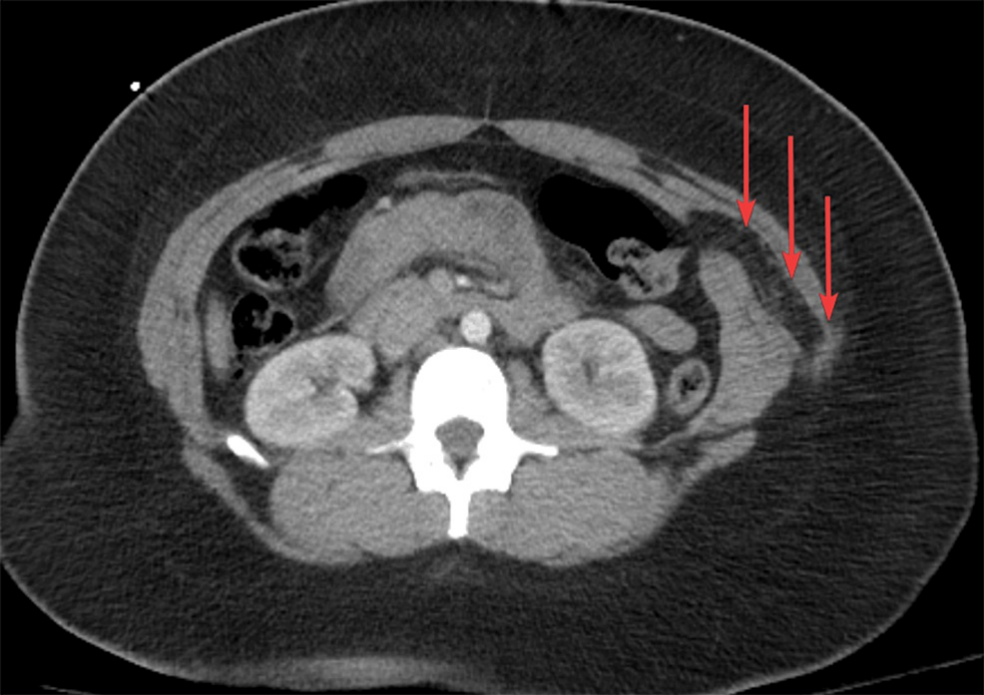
Contrast-enhanced computed tomography, axial view of the penetrating wound through the left-sided abdominal musculature and into the peritoneum with herniation of the omentum. The herniated omentum is marked with arrows.
